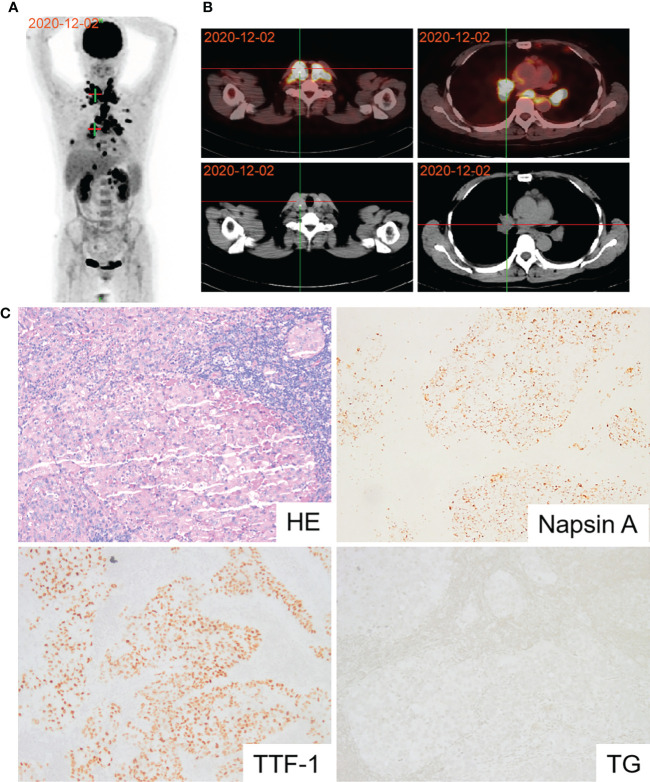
Diagnosis of lung adenocarcinoma using PET and pathology. (A) PET-CT scan revealing active metabolic activity in the right lower lobe of the lung, multiple lymph nodes, adrenal gland, pleura and thyroid. (B) PET-CT image of thyroid and lung lesions. (C) Pathological image of lymph node biopsy confirming lung adenocarcinoma.
